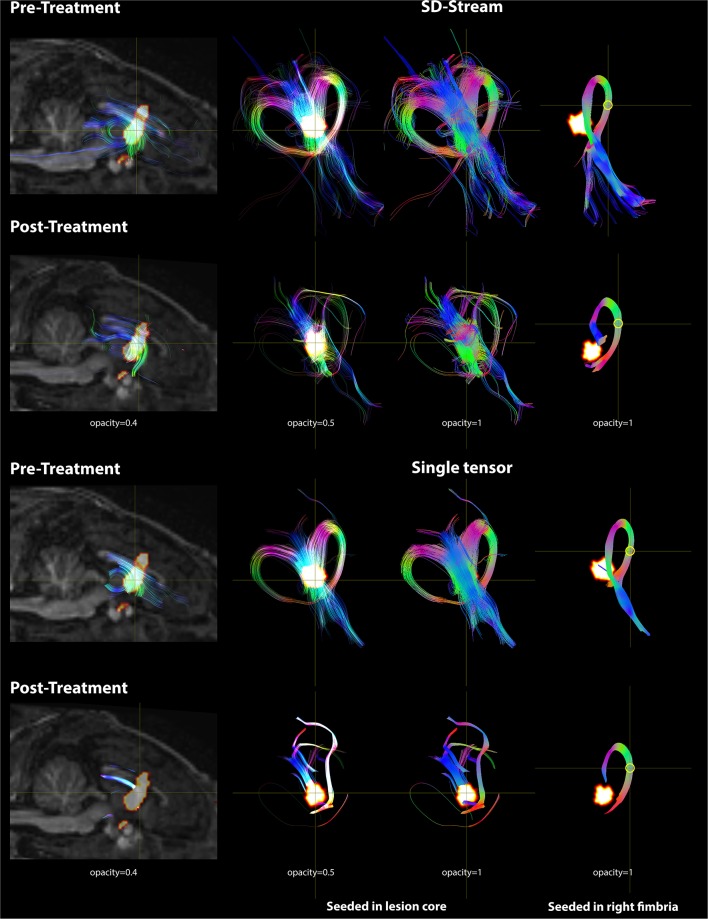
DWI tractography seeded in the lesion core and right fimbria is presented with both the FOD model using SD-Stream and the single tensor model. Left: mid-sagittal T1w image overlaid with the lesion core (seed region) and the tracts generated based on that seed (with opacity of 0.4). Middle: tracts seeded in the lesion core in 3D space with opacity of 0.5 and 1, respectively. Right: tracts seeded in the right fimbria (yellow circle) in 3D space. Tracts are colored by orientation (red, right–left; green, superior–inferior; blue, anterior–posterior).
